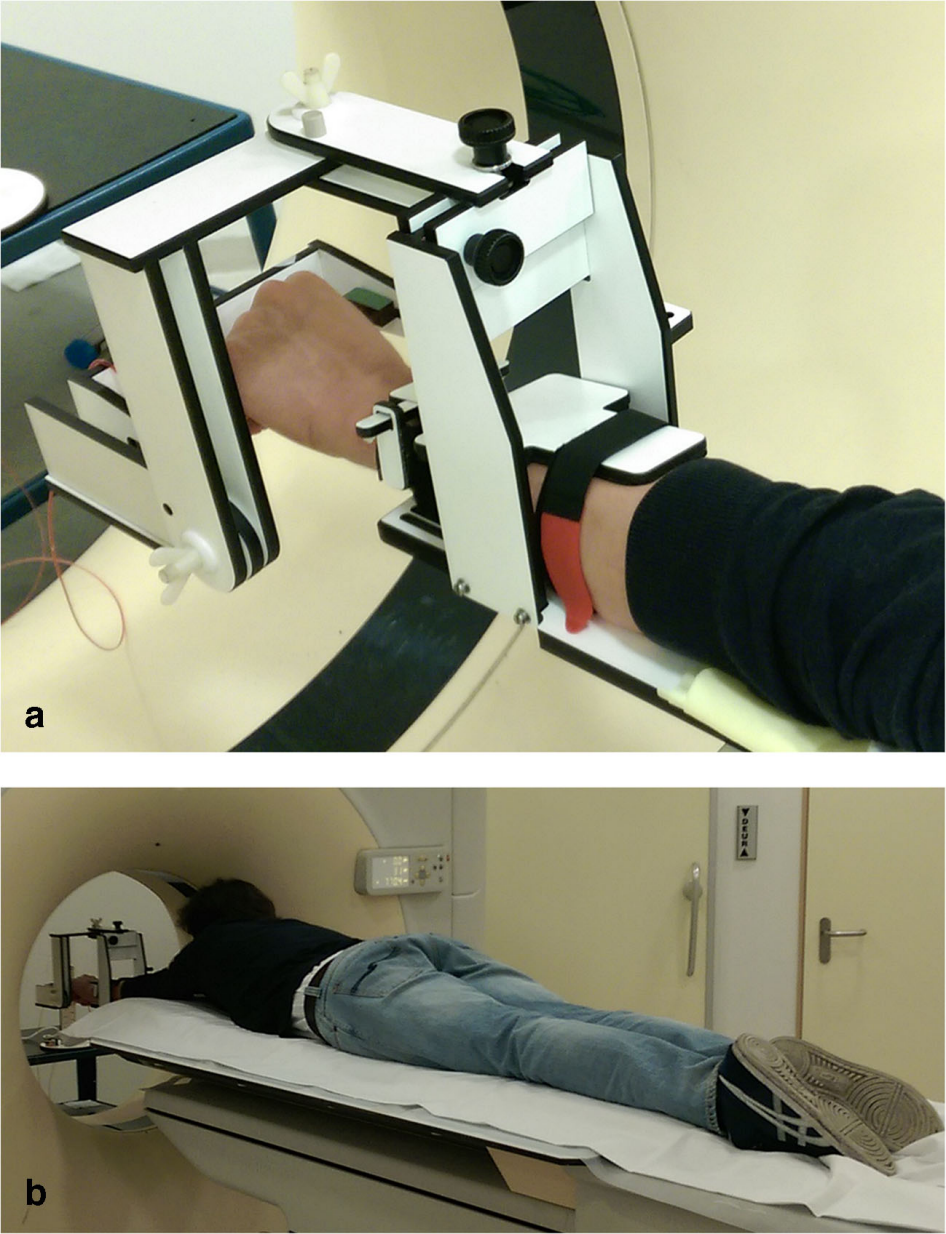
a Custom-made device to guide the hand and wrist during the 4D-CT recordings. b Positioning of a participant during 4D-CT recordings
